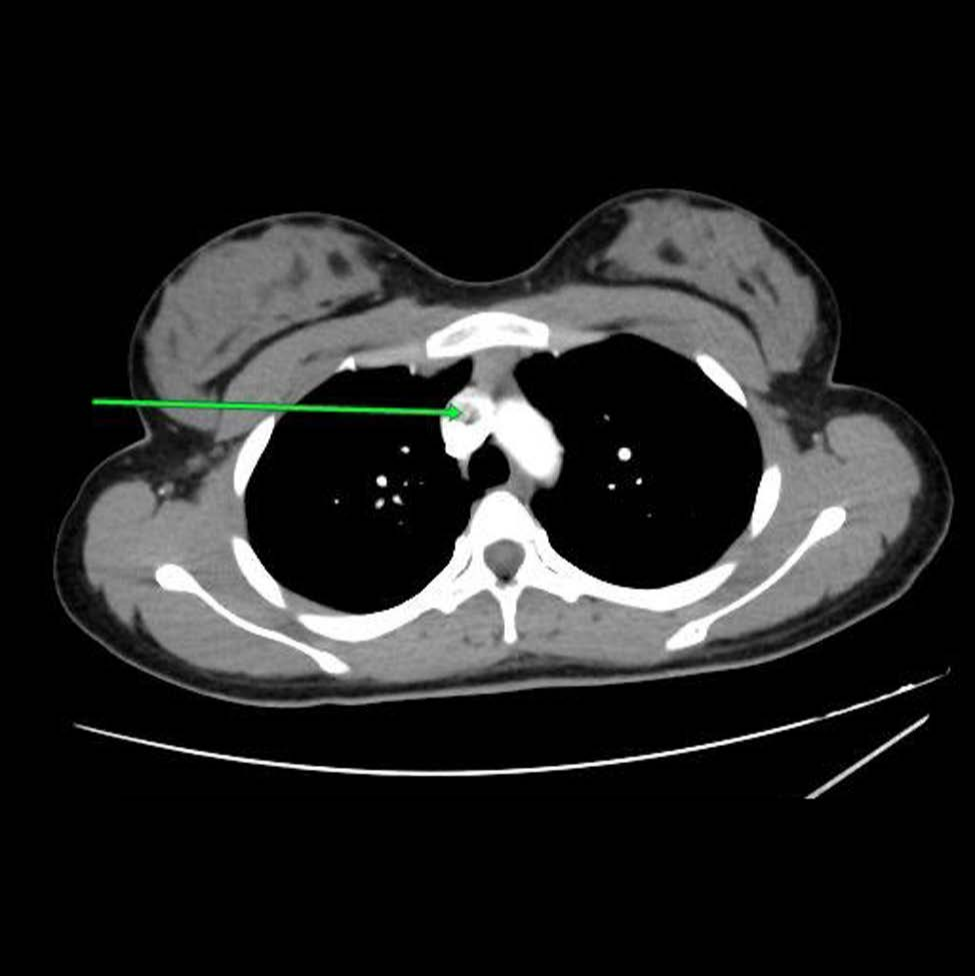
Axial section of a computed tomography pulmonary angiogram at the level of the main pulmonary vessels showing partial filling defects in the main right pulmonary artery as highlighted with the arrow.
